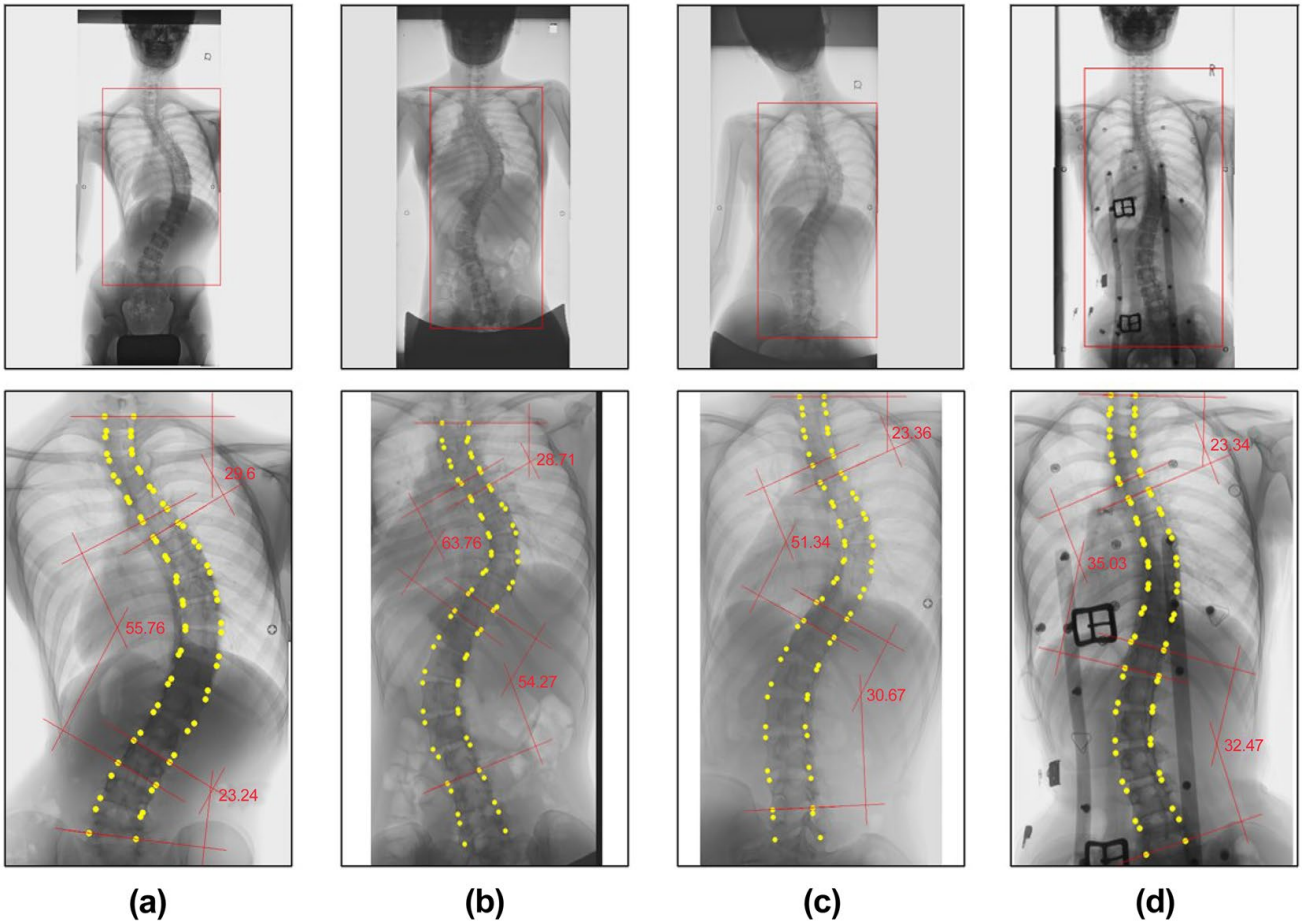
Results of CA measurement using the proposed method. The upper row shows the detected ROI indicated by a rectangle on the image, and the lower row shows the CA measurement results. Each column shows examples of (A) standing, (B) supine, (C) bending, and (D) wearing-brace X-ray images.
